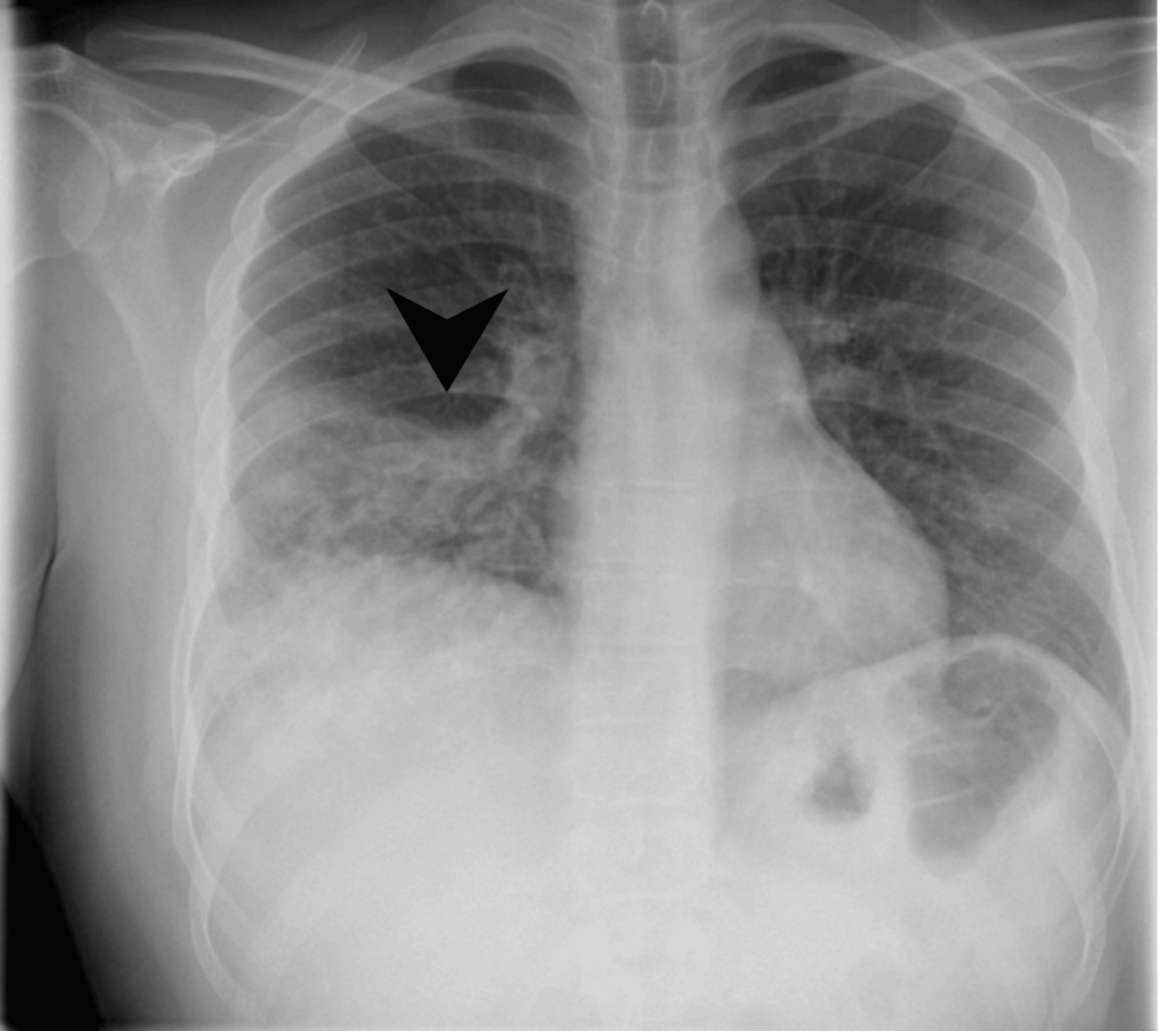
Posteroanterior chest radiograph showing right lower lobe consolidation (arrowhead) with a focal area of increased opacity in the right lower lung zone.
